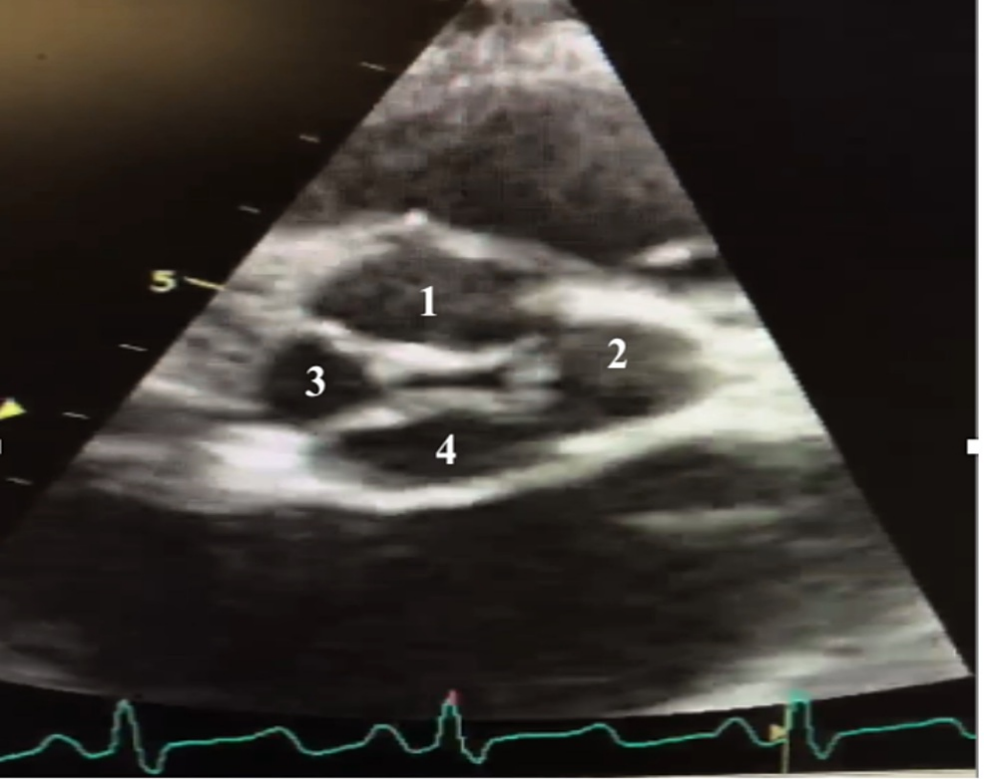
Transesophageal echocardiogram taken two days after admission showing quadricuspid valve (1-4)
